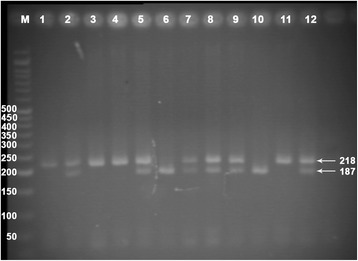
Agarose gel electrophoresis for the rs5888 polymorphism of scavenger receptor class B type 1 (SCARB1) gene. The 218-bp PCR product of SCARB1 rs5888 (C > T) was digested by Hin1I (BsaHI) restriction enzyme. The C allele codes for the presence of the Hin1I site and the T allele codes its absence. The wild-type CC variant produced two fragments with 187 bp and 31 bp while heterozygote CT produced 3 fragments of 218 bp, 187 bp, and 31 bp. The mutated homozygous variant TT produced one fragment of 218 bp. The 31 bp fragment was not visible in the gel due to its fast migration speed. M, 50 bp marker ladder; Lanes 6 and 10, CC genotype; Lanes 2, 5, 7, 8, 9, and 12, CT genotype; Lanes 1, 3, 4, and 11, TT genotype
